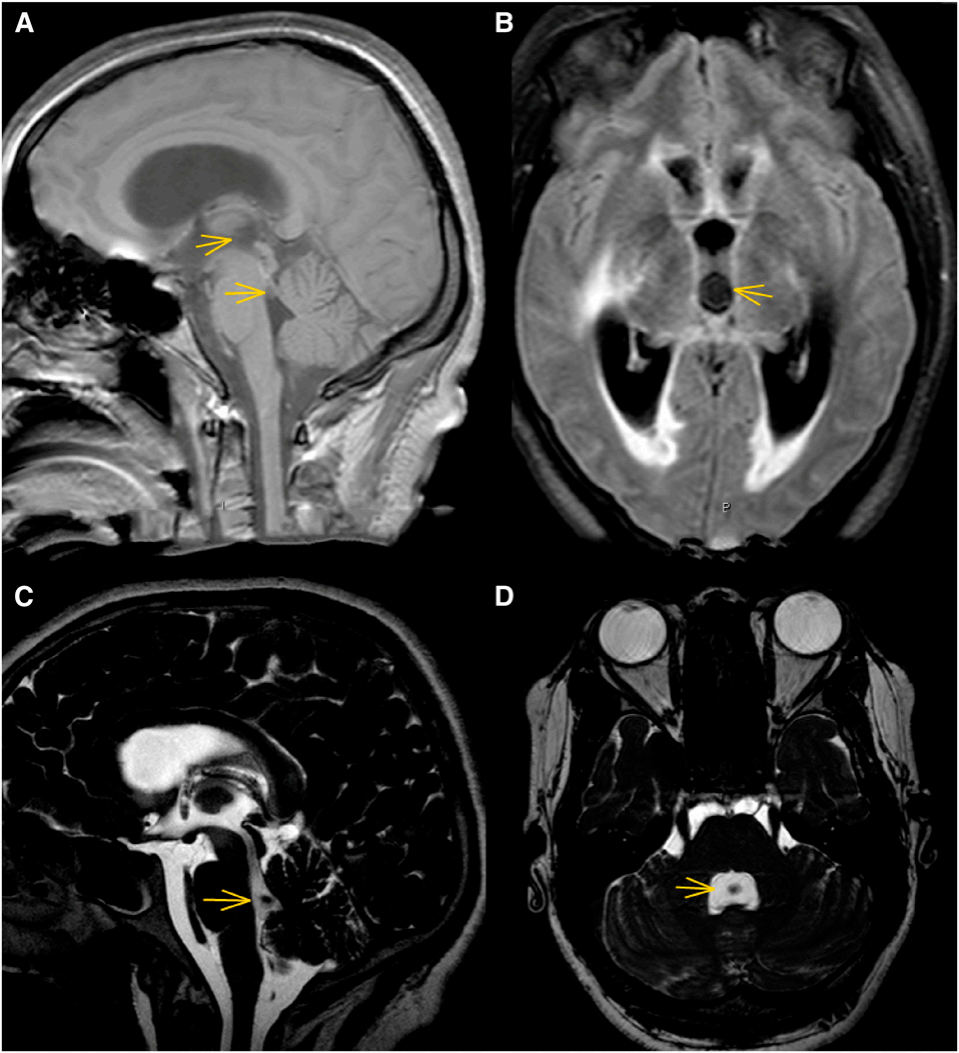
MRI imaging of the case. Panels (A) and (B) are imaging performed on the day of presentation, February 7, 2011, panel (C) on the day after presentation, February 8, 2011 and panel (D) on the day of admission to National Institutes of Health on June 2, 2011. Panel (A) is a sagittal T1 weighted fast field Echo (FFE) image revealing a barely visible third ventricle cyst showing [better seen in the axial view in Panel (B)] and part of the cyst in the process exiting the aqueduct into the fourth ventricle. Arrows delineate the cyst in the third and fourth ventricles. Panel (B) is an axial fast-attenuated inversion recovery image showing acute hydrocephalus with transependymal flow and a third ventricular cyst (arrow). Panel (C) is a cerebrospinal fluid-driven equilibrium radiofrequency reset pulse image demonstrating the scolex now fully situated in the fourth ventricle. Panel (D) is an axial balanced FFE image on June 2, 2011 (balanced fast field echo) image showing a cyst occupying the fourth ventricle (arrow) with a calcified scolex seen as a void in the middle of the cyst. This figure appears in color at www.ajtmh.org.
